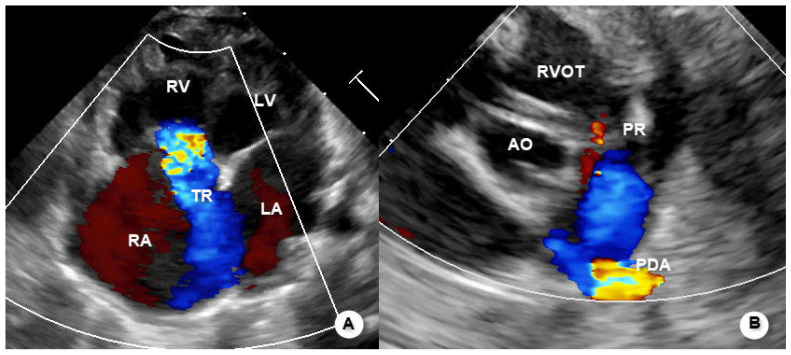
Representative echocardiographic images of functional pulmonary atresia. (A) Apical four-chamber view demonstrating enlargement of the right atrium (RA) and right ventricle (RV) with severe tricuspid regurgitation (TR). (B) Parasternal short-axis view showing absence of antegrade pulmonary valve flow, with minimal pulmonary regurgitation (PR); pulmonary arterial blood flow is supplied predominantly via the patent ductus arteriosus (PDA). Abbreviations: RA, right atrium; RV, right ventricle; LA, left atrium; LV, left ventricle; RVOT, right ventricular outflow tract; AO, aorta; TR, tricuspid regurgitation; PR, pulmonary regurgitation; PDA, patent ductus arteriosus.
